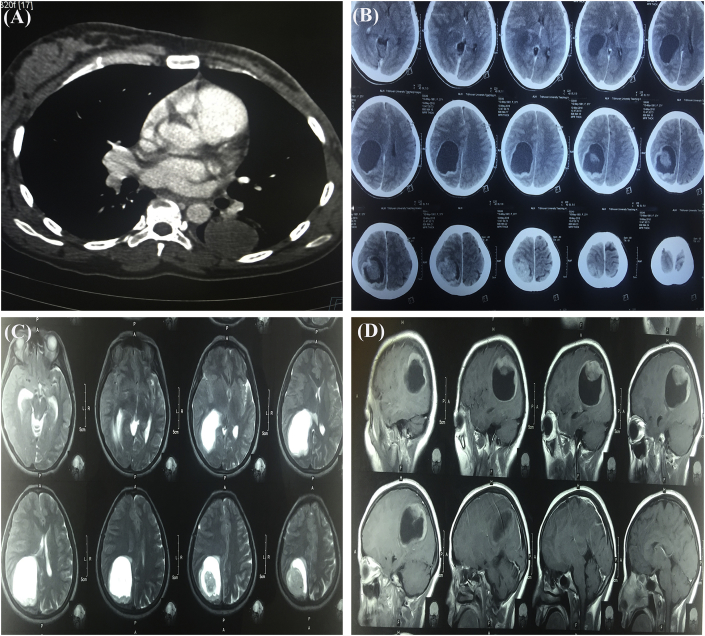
(a) Well defined round soft tissue density lesion of +40HU(Hounsfield unit) in posterobasal segment of left lower lobe of lung (b) CT scan of head showing well capsulated cystic lesion with heterogenously enhancing eccentric mural nodule with significant midline shift (c & d) T2 and T1 weighted MRI with Gadolinium contrast, respectively of head with enhancing wall, mural nodule and significant midline shift.
